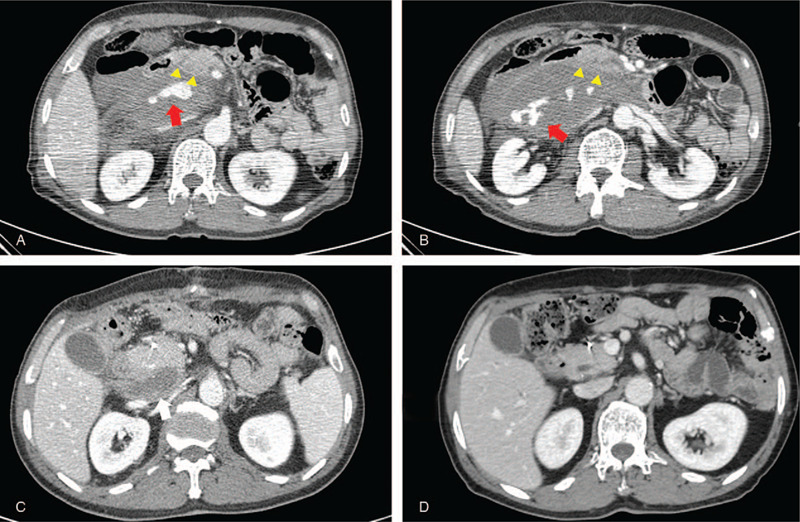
Case 1: a 64-year-old man was admitted to our trauma center after falling down from a height of 4 m. A, Multidetector computed tomography (MDCT) scan revealed as large amount of retroperitoneal hematoma at the posterior aspect of the pancreas head with internal extravasation of contrast media suggesting active bleeding (red arrow). Although there was a subtle low-density lesion suggesting mild contusion at the head of the pancreas, the shape of pancreas (yellow arrow head) and biliary duct were relatively well-preserved. B, Moreover, active bleeding (red arrow) was observed on the axial image scanned at the lower level, and there was no pneumoperitoneum or abnormal thickening of the duodenal wall (yellow arrow head). C, Follow-up MDCT scan 10 days after transcatheter arterial embolization (TAE) revealed a low-density lesion indicating an old hematoma (white arrow) at the posterior aspect of the pancreas head, and this lesion was smaller than before TAE. D, At 7 months follow-up computed tomography (CT), the hematoma had disappeared completely.
